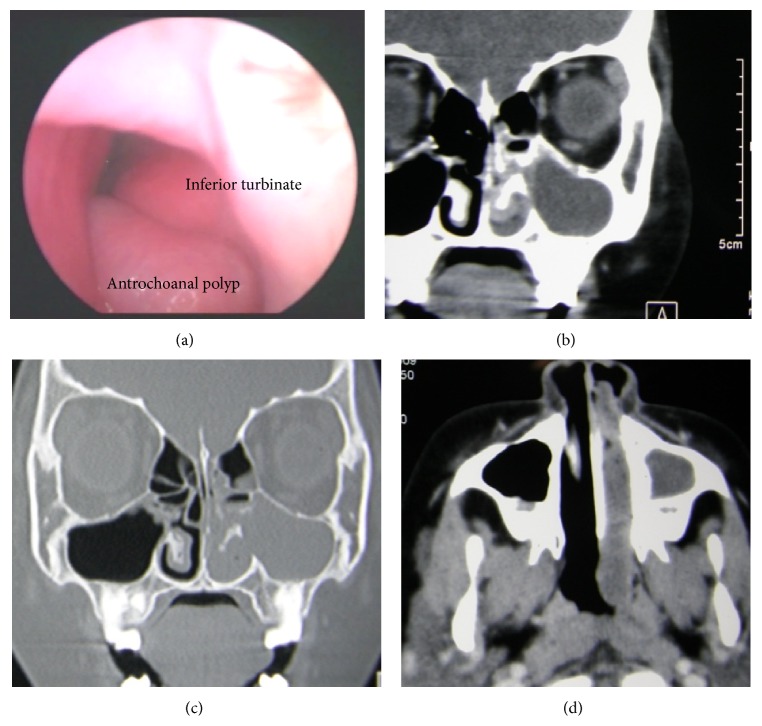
(a) Left nasal cavity endoscopic view showing antrochoanal polyp at inferior meatus CT scans. (b) Coronal view, soft tissue window showing cystic component in the maxillary antrum. (c) Coronal view, bone window showing defect of medial maxillary wall below the inferior turbinate. (d) Axial view, soft tissue window; polyp extending into the nasopharynx.
